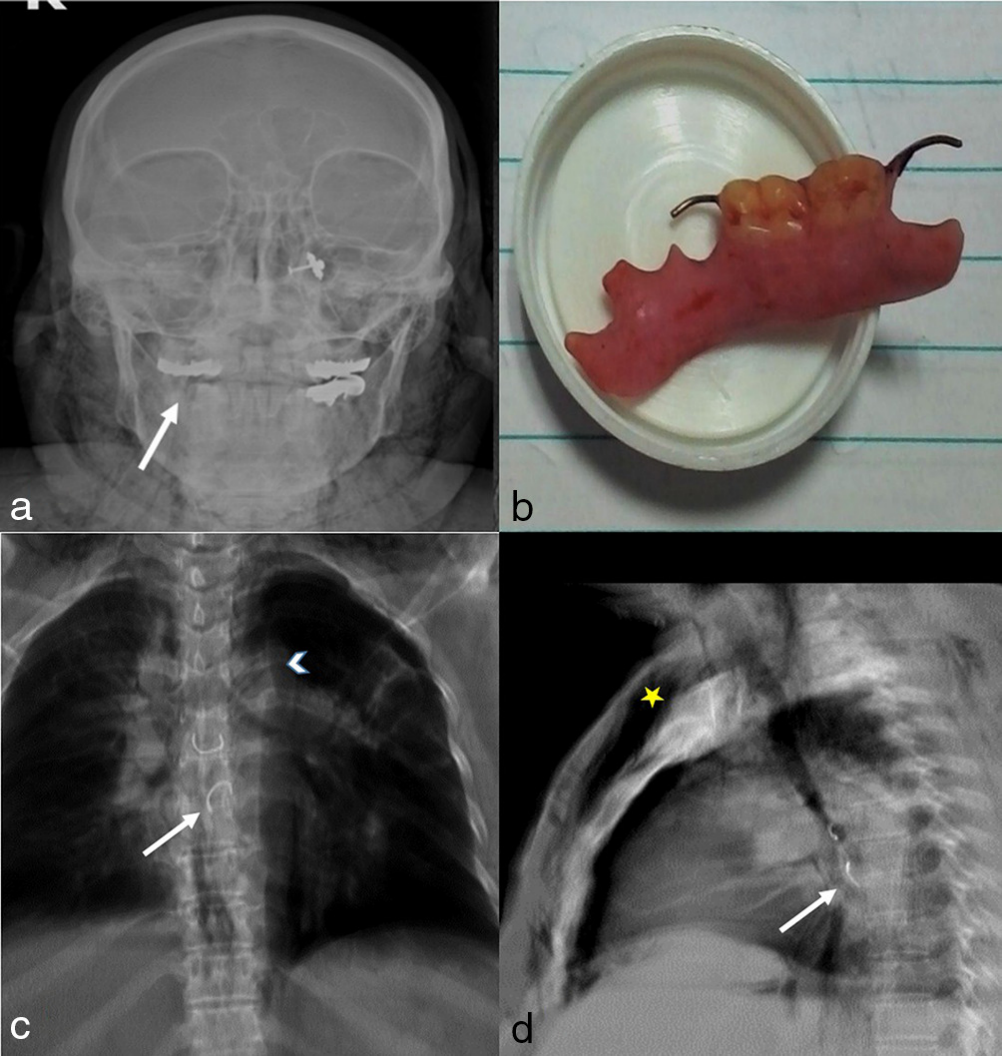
(a) Skull radiograph, frontal view, showing the missing denture from the right lower jaw (white arrow). (b) Thoracoscopically removed partial denture. (c, d) Anteroposterior and lateral view chest radiographs showing a metallic density foreign body in the retrocardiac region (white arrows), pneumomediastinum (arrowhead) and subcutaneous emphysema (yellow star).
